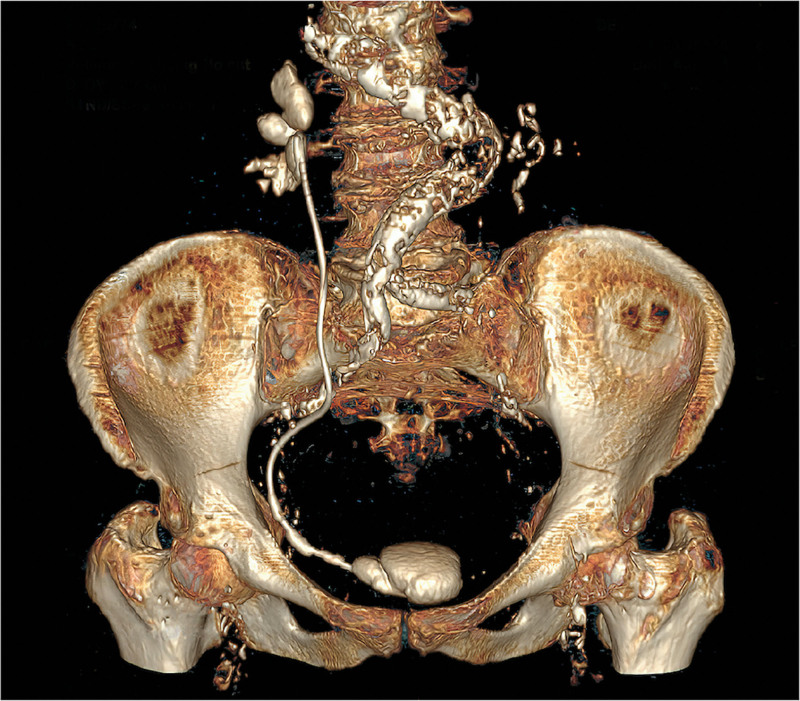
Three-dimensional reconstruction of CT images showing an encrusted DJ stent. CT = computed tomography, DJ = double J.
